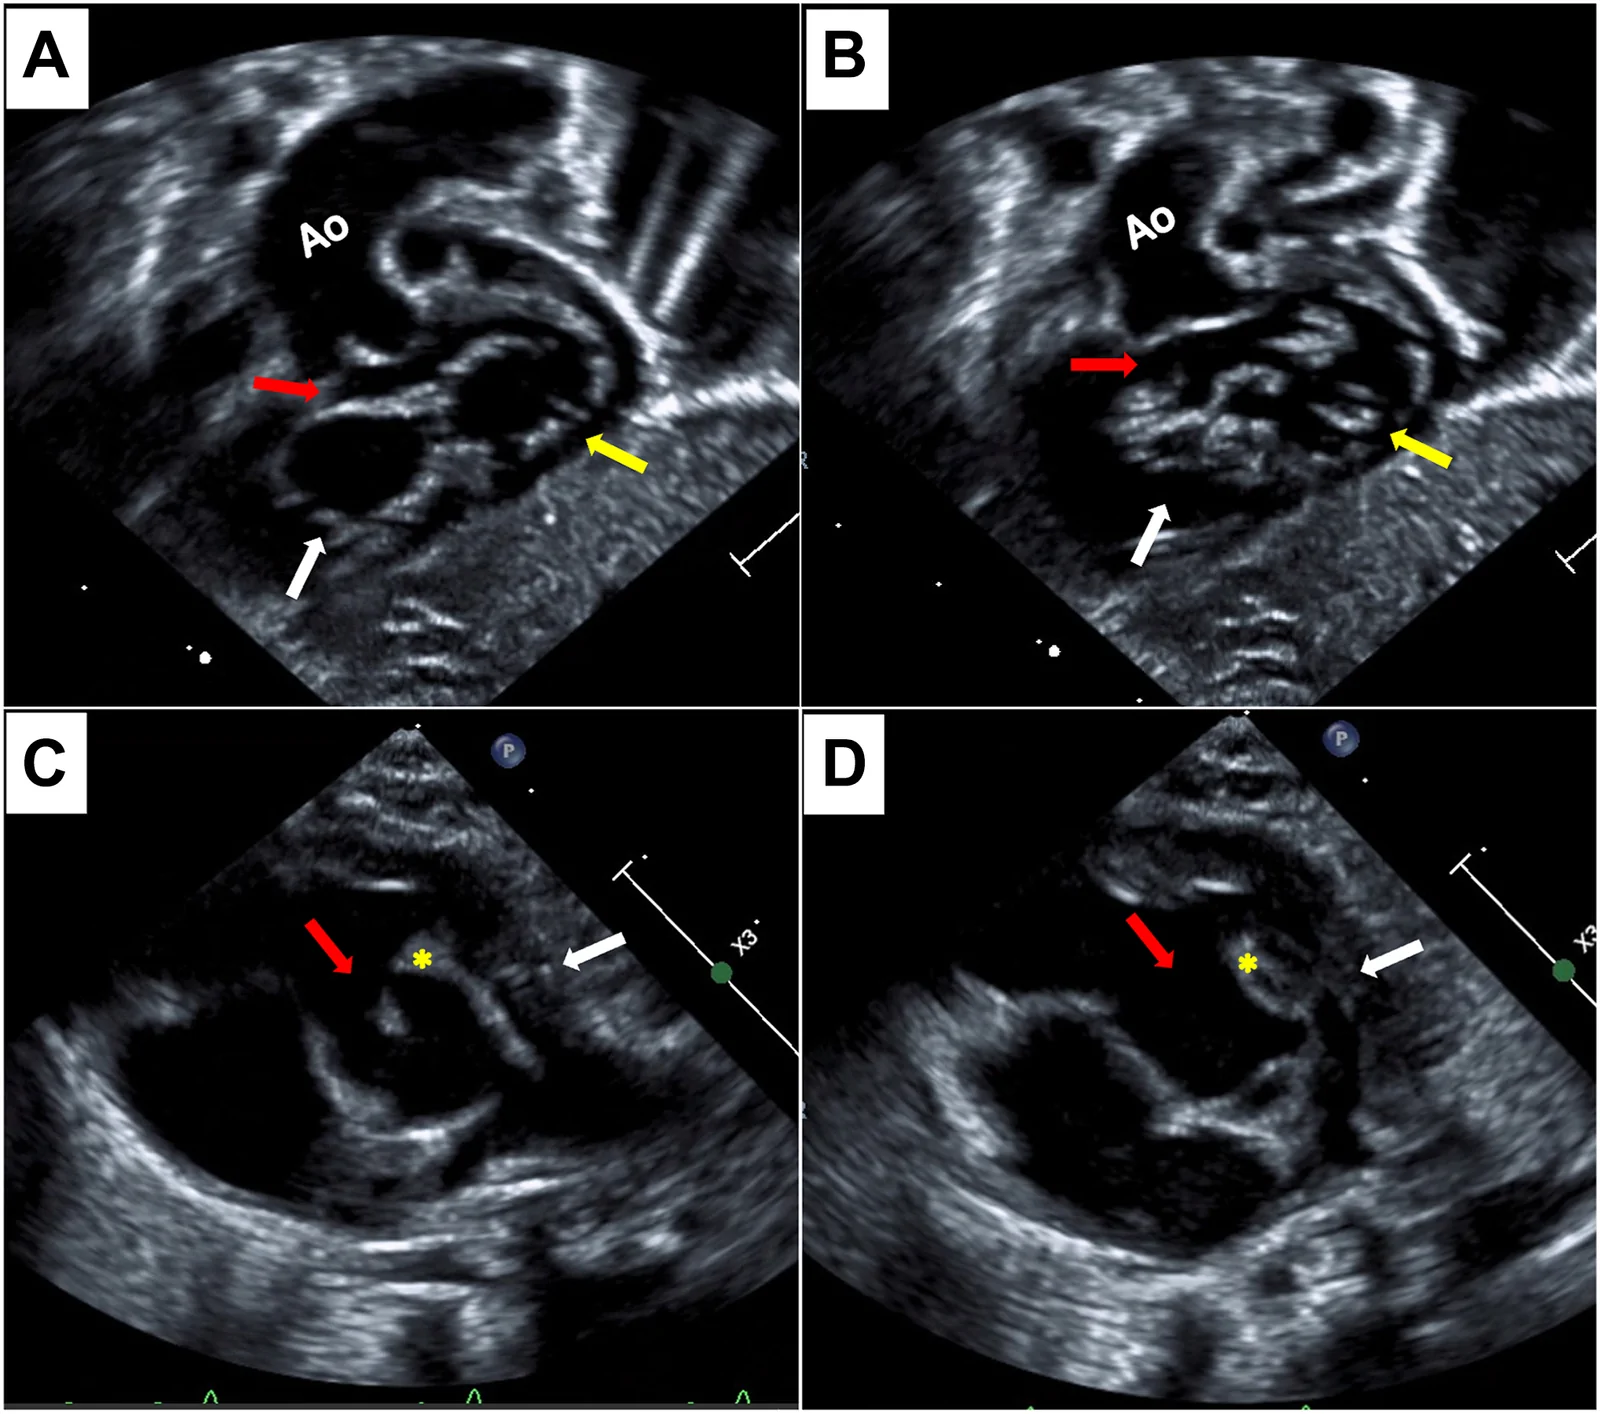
Preoperative 2D TTE, subcostal left anterior oblique (A, B) and parasternal short-axis (C, D), diastolic (A, C) and systolic (B, D) views, demonstrates the aorta (Ao) overriding the ventricular septum, the large VSD *(red arrows)*, and a common AVV divided into 2 separate orifices with the right AVV (*white arrows* in A, B) and left AVV (*yellow arrows* in A, B) with a trifoliate appearance characteristic of a common AVV, as well as anterior malalignment of the conal septum (∗) with subsequent narrowing of the RVOT and plate-like atresia of the pulmonary valve (*white arrows* in C, D) consistent with TOF with pulmonary atresia.
